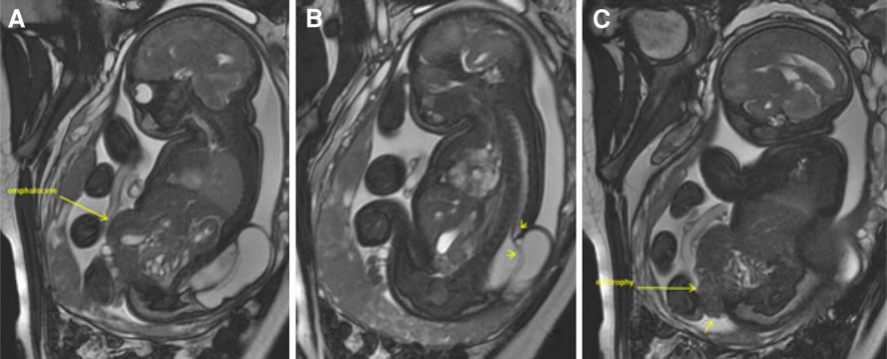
Prenatal MRI of a patient with cloacal exstrophy showing the omphalocele (a), neural tube defect (b) and bladder exstrophy (c)
